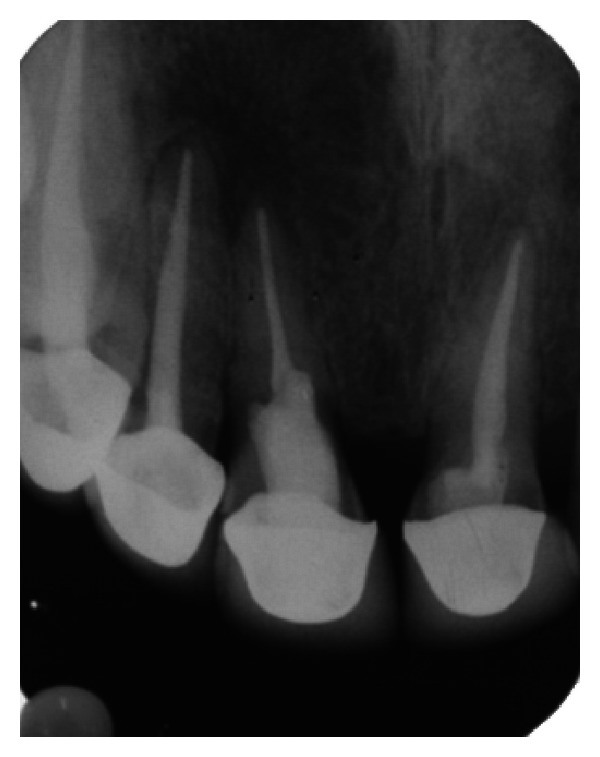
18-month followup IOPA radiograph.
